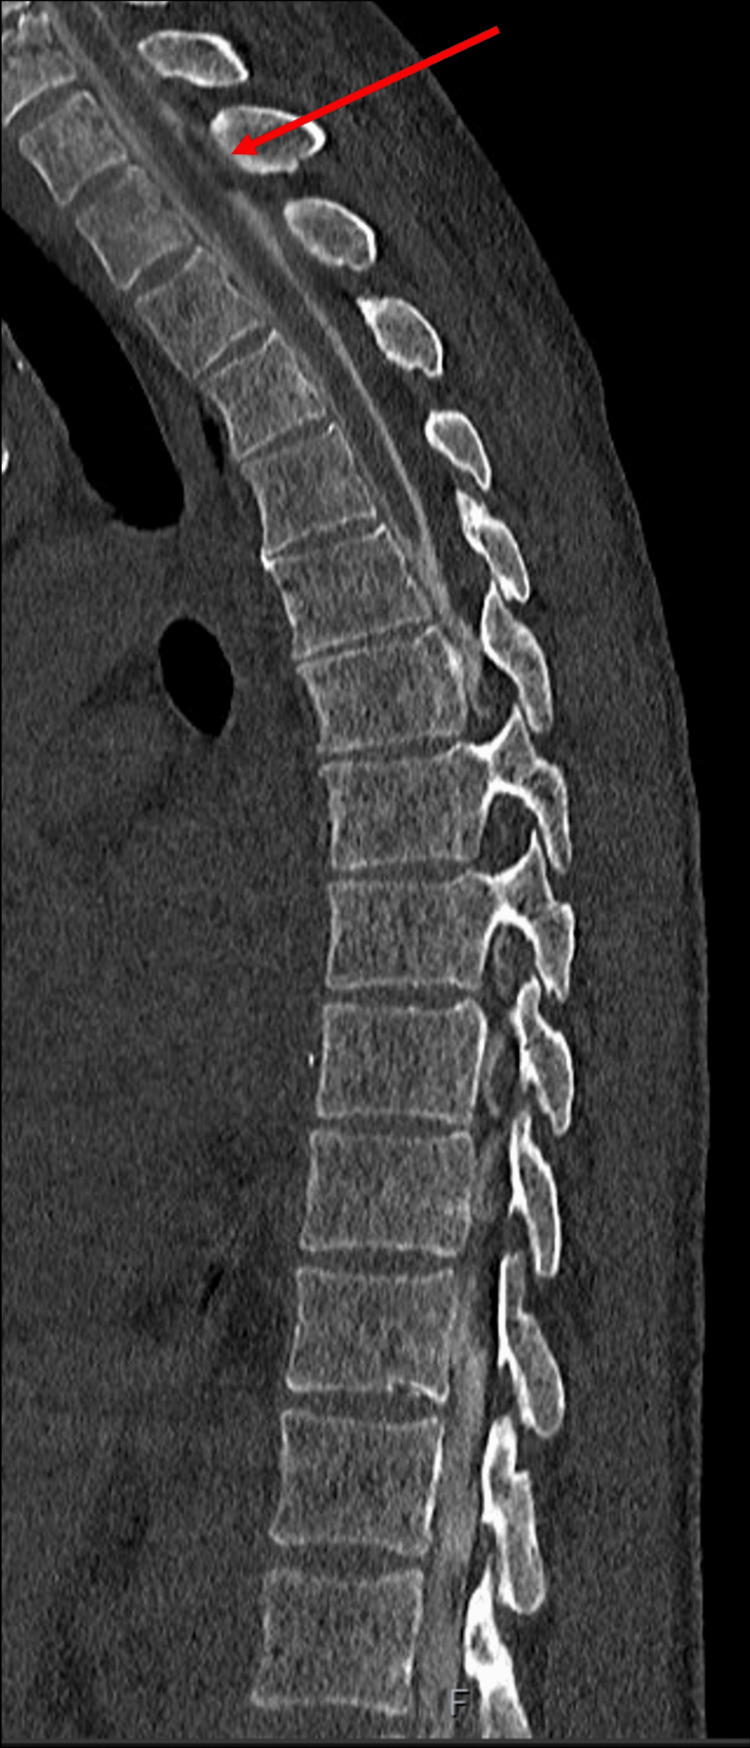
Dynamic CT myelogram showing a thin longitudinal extradural fluid collection along the dorsal aspect of the thecal sac in the cervical and upper thoracic regions
